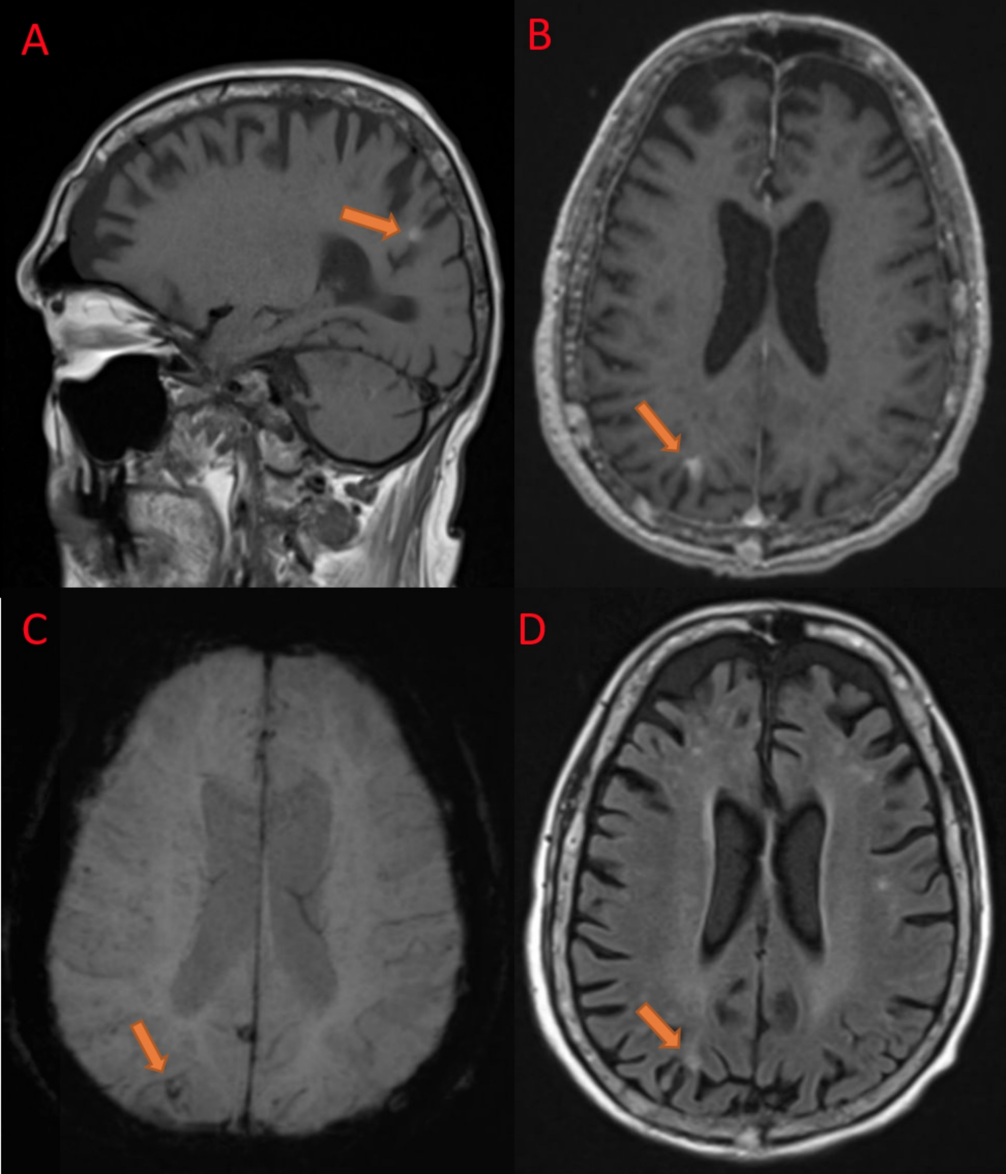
Magnetic resonance imaging of the Brain A) Sagittal T1 weighted image showing hyperintensity in the right parietal region (arrow), B) Axial T1 weighted post-contrast image showing no significant enhancement compared to T1-weighted image (arrow), C) Axial susceptibility-weighted Image (SWI) showing small blooming artifact in the same location (arrow), and D) Axial T2-weighted fluid attenuation and inversion recovery (FLAIR) scan showing hyperintensity (arrow) (seen in late subacute hemorrhage).
